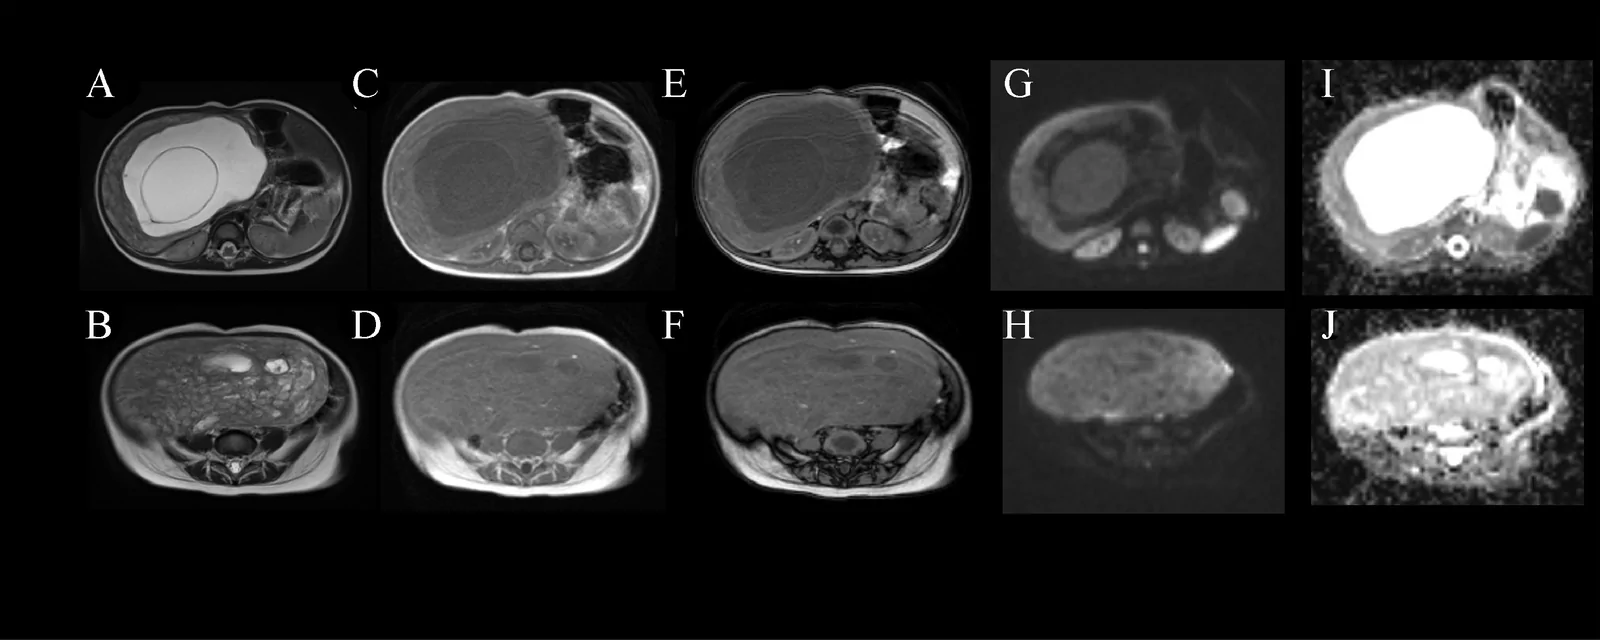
MRI. (A and B) Axial T2-weighted images acquired using the BLADE technique, a motion-resistant radial k-space sampling method; (C and D) axial T1-weighted in-phase images; (E and F) axial T1-weighted opposed-phase images; (G and H) diffusion-weighted images obtained at a b value of 800 s/mm²; and (I and J) apparent diffusion coefficient maps. The MR images were obtained at different axial levels to best demonstrate the cystic and solid components of the tumor. The cystic component shows low signal on T1-weighted imaging (T1WI) and high signal on T2-weighted imaging (T2WI). A solid component demonstrates a heterogeneous signal: low intensity on T1WI and mixed low-to-high signal on T2WI. No restricted diffusion is evident on the diffusion-weighted images or apparent diffusion coefficient maps.
